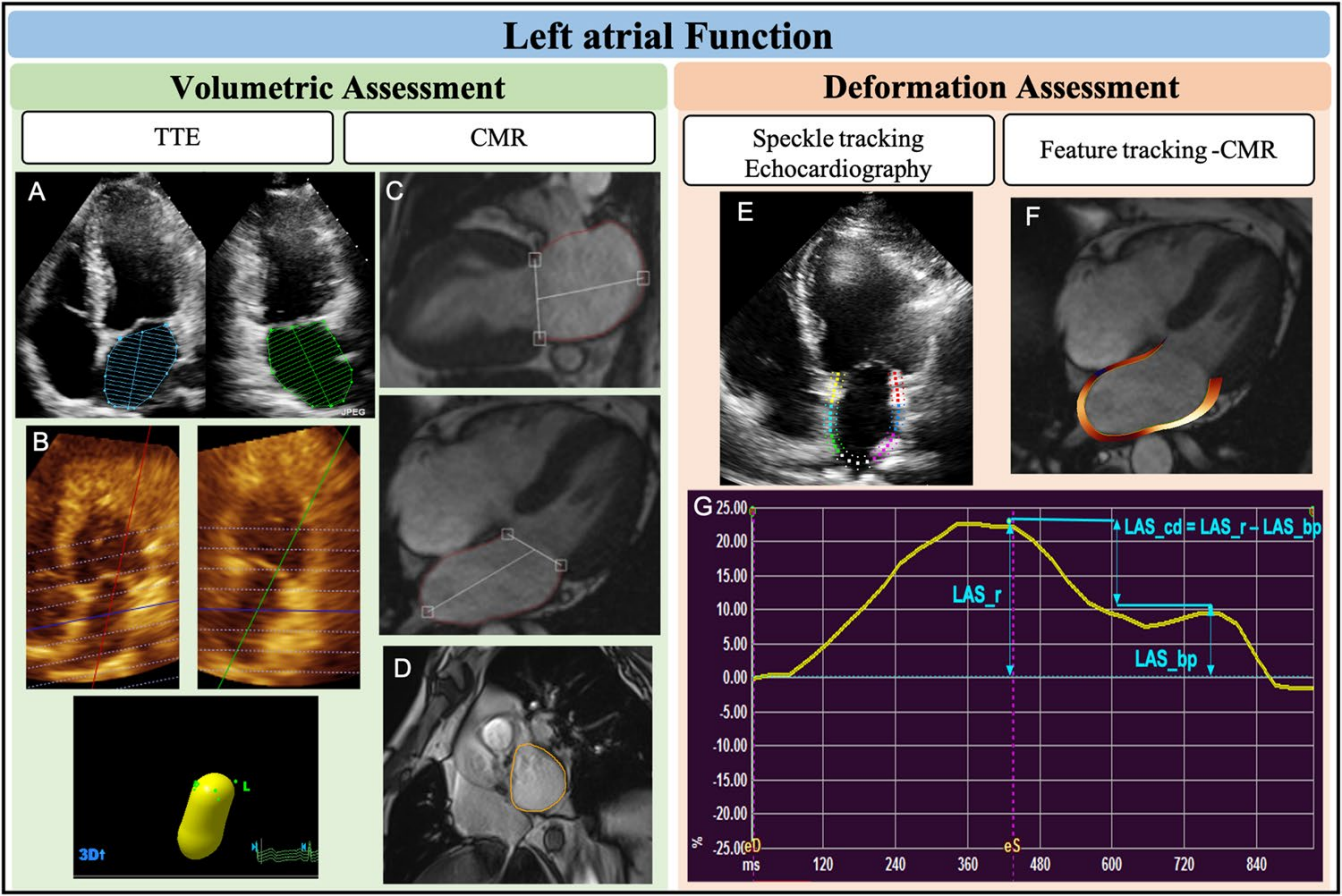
Imaging assessment of left atrial function. LA volumetric assessment using Transthoracic echocardiography (TTE) include biplane disk method (A) and 3D method(B), and using CMR include biplane area length method (C), and short axis stack method (D). LA deformation assessment using Speckle tracking echocardiography (E), and feature tracking on CMR (F). An example of LA strain curve and the measurements corresponding to LA phases (G). LAS_r = LA strain at reservoir, LAS_cd = LA strain at conduit, LAS_bp = LA strain at booster-pump phase
